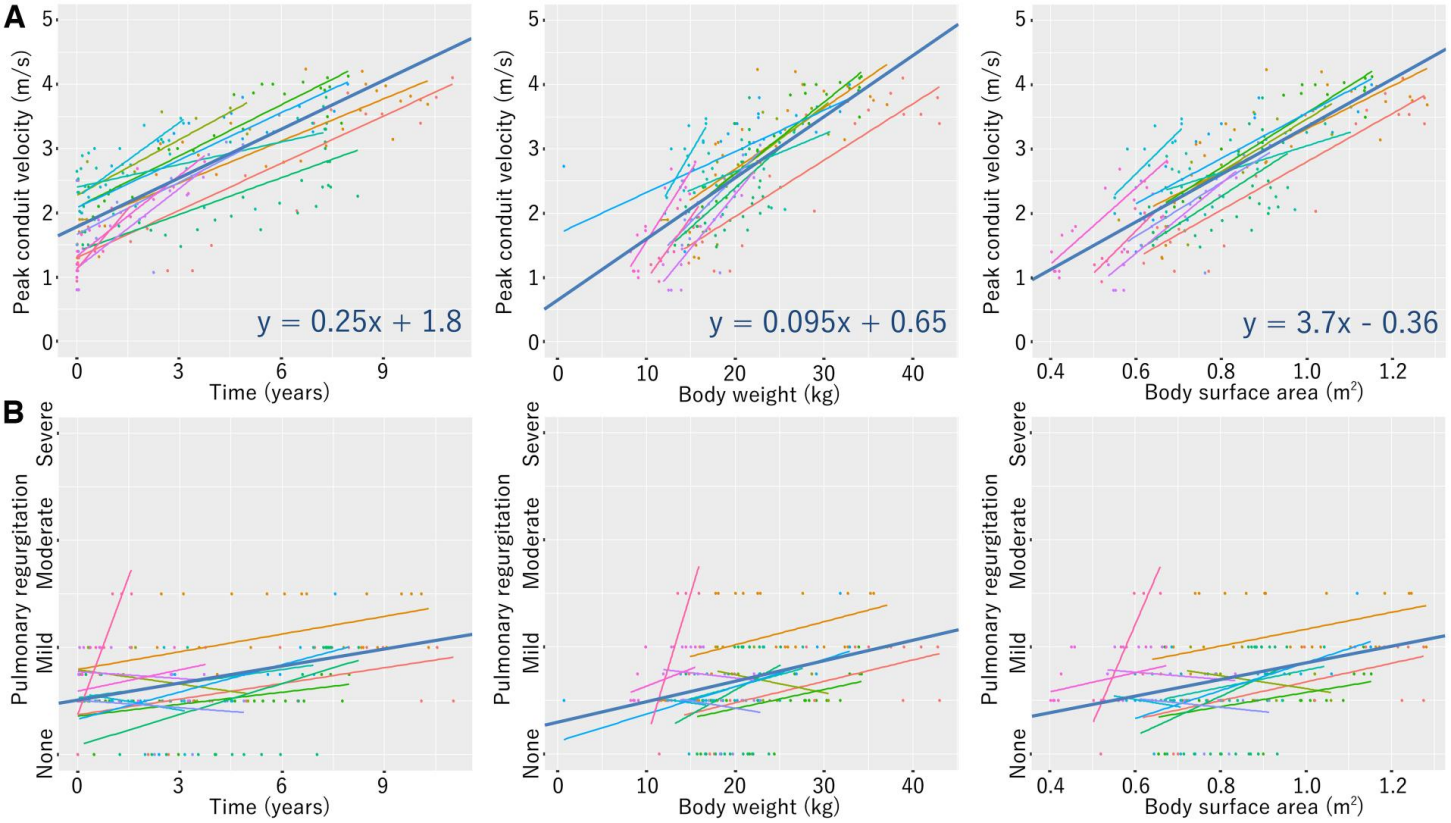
Linear mixed-effects models of echocardiographic peak conduit velocity (A) and pulmonary regurgitation (B) in patients with the 18-mm conduit
